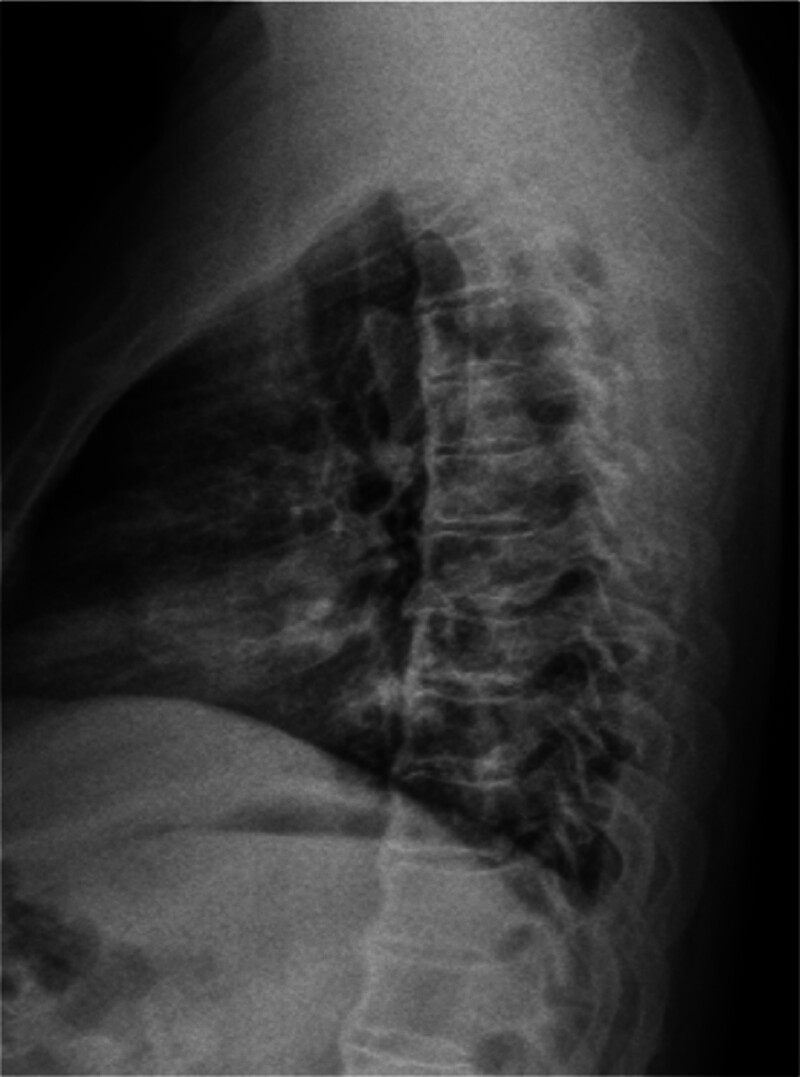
Lateral plain film of thoracic vertebra: it can be seen that there is osteoporosis and bone degeneration.
